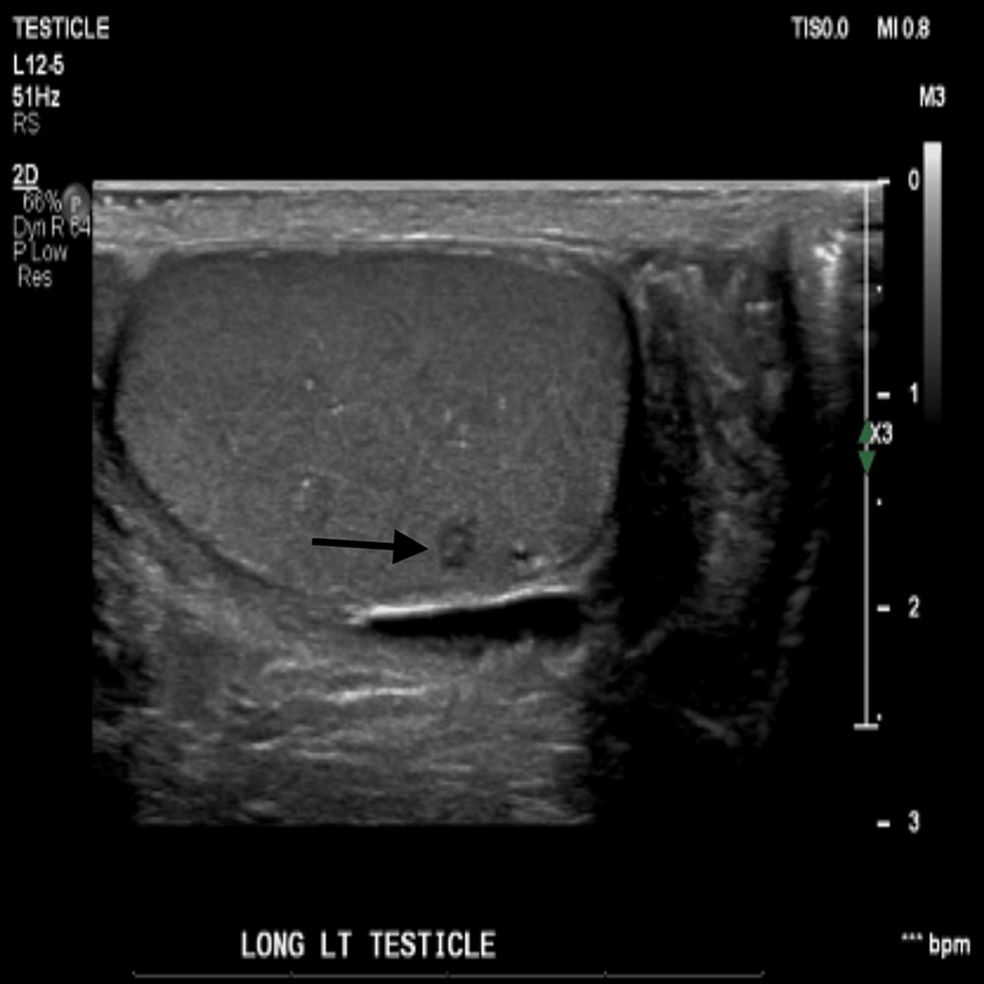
Scrotal ultrasound of the left testicle, longitudinal axis, which reveals an area of hypoechoic focus consistent with granuloma.
